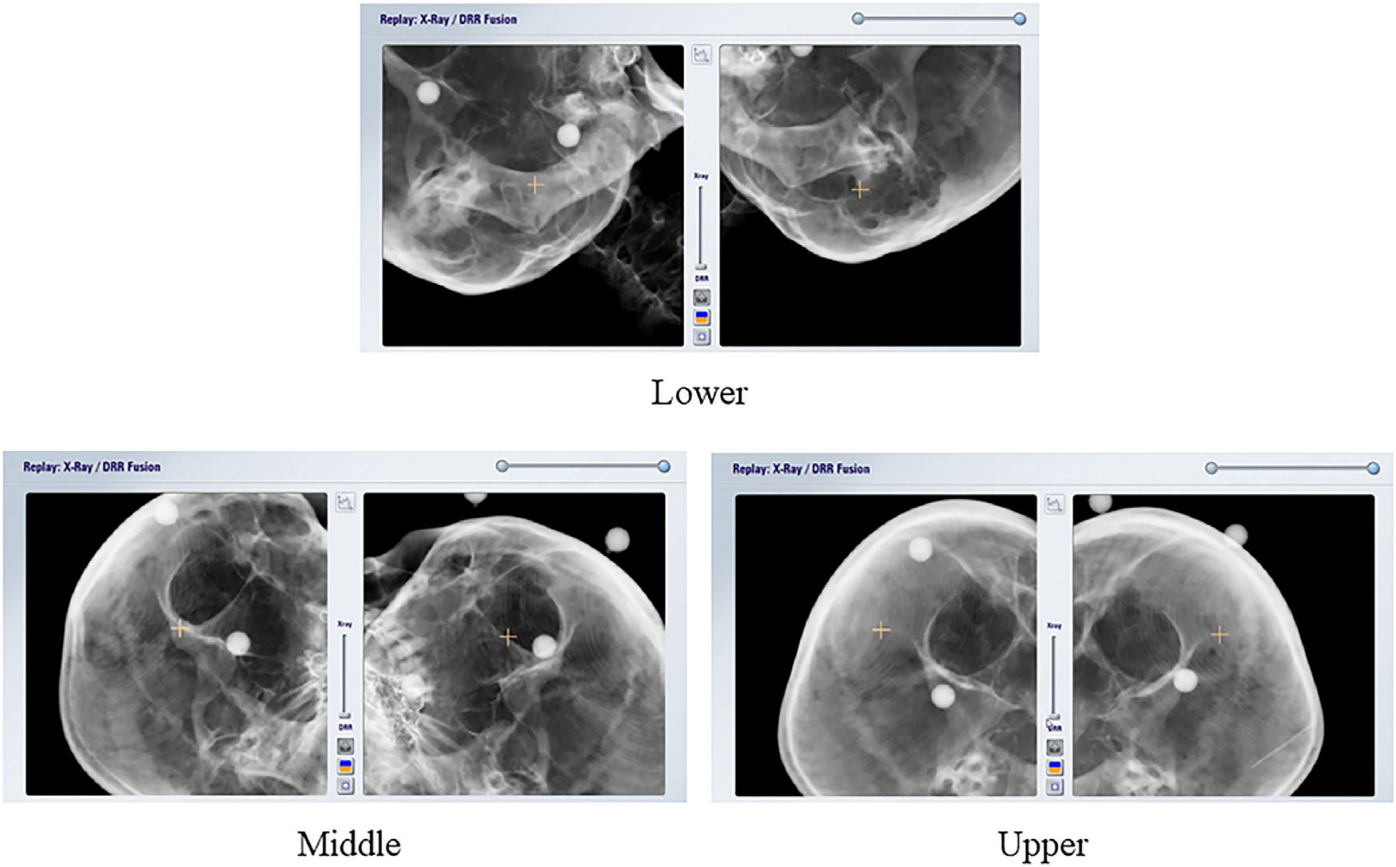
Three classifications of brain metastases according to the region of occurrence (lower, middle, and upper) Abbreviation: DRR, digitally reconstructed radiograph.
